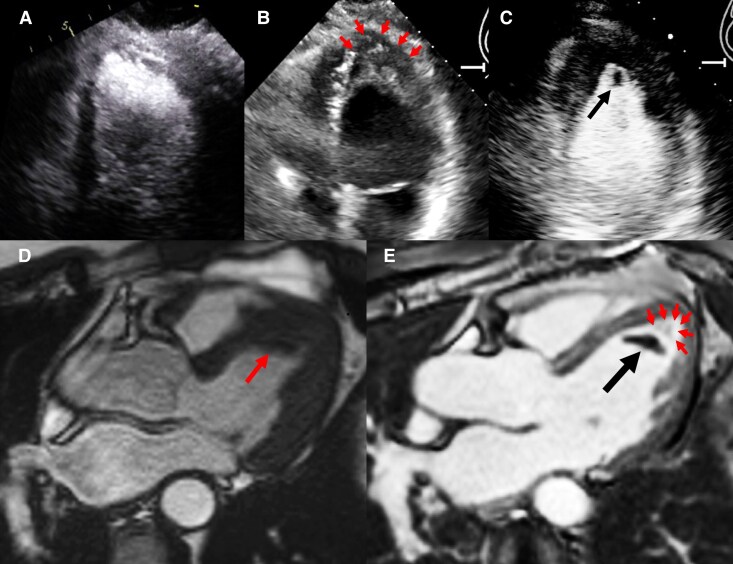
Multimodal cardiac imaging demonstrating development of endomyocardial fibrosis. Red arrows indicate endomyocardial fibrosis/thickening; black arrows indicate LV apical thrombus. (*A*) Contrast-enhanced TTE obtained 3 years prior to current presentation showing normal LV apex without endomyocardial thickening or thrombus, with normal LVEF and wall motion. (*B*) Repeat TTE without contrast at the current presentation showing increased apical wall thickness (red arrows). (*C*) Contrast-enhanced TTE confirming apical thrombus (black arrow). (*D*) Cardiac MRI cine confirming thickening of apical segments (red arrow). (*E*) Late gadolinium enhancement imaging showing dense fibrosis (red arrows) in the endomyocardial layer of the apical segments and confirming LV apical thrombus (black arrow).
